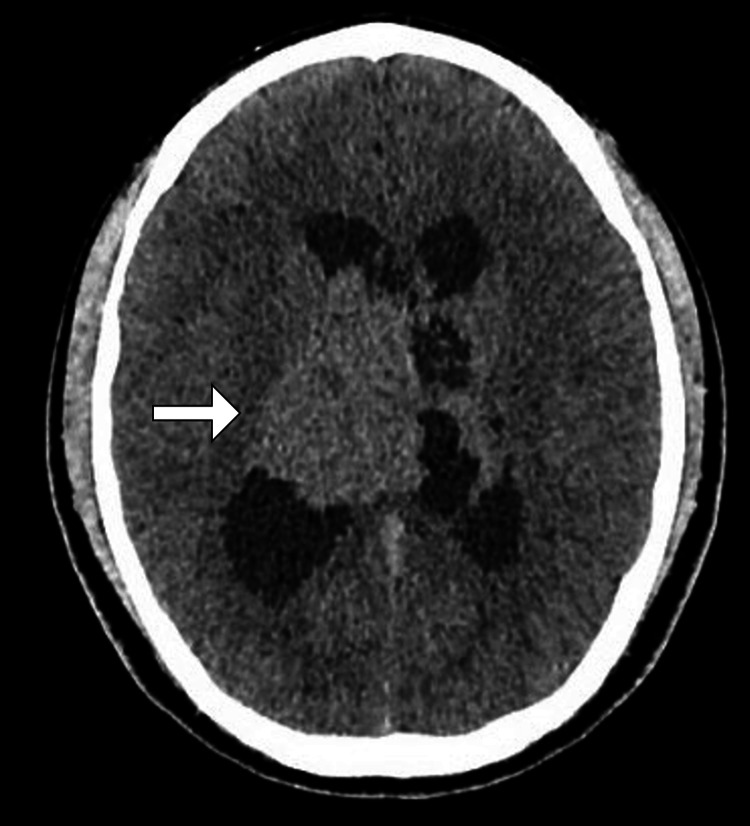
CT image of the brain Axial CT image of the brain demonstrating a slightly hyperdense lesion (arrow) within the right lateral ventricle with associated hydrocephalus. CT, computed tomography
